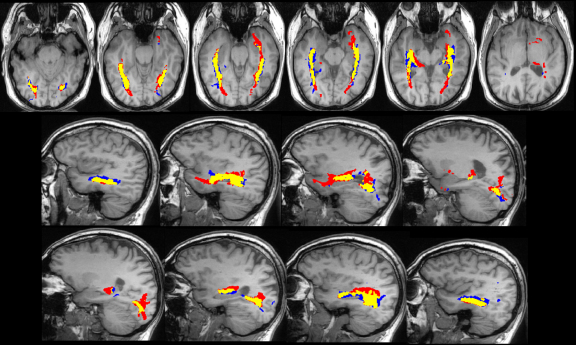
Optic radiation identified by Method 1 only (blue), Method 2 only (red) and both methods (yellow) in a control subject, overlaid on the T1-weighted image. The images are shown in radiological convention with axial slices from inferior to superior and sagittal slices from left to right.
